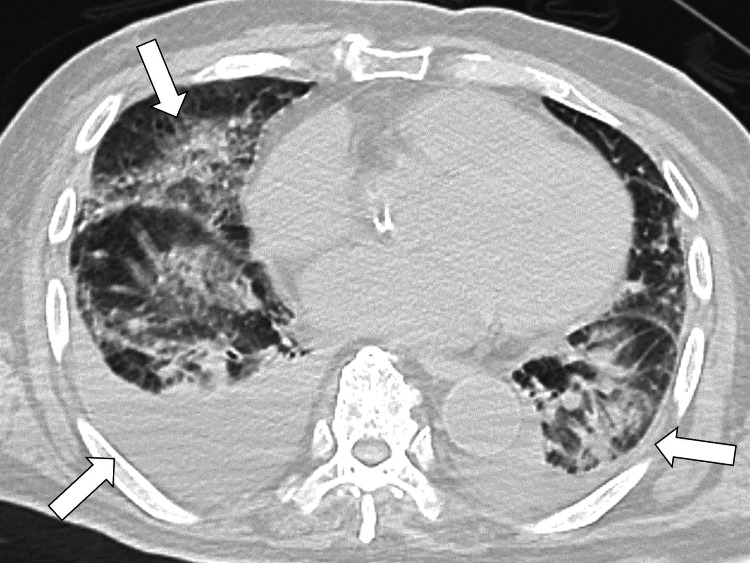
CT of the chest showing infiltrative shadows and pleural effusions in bilateral lungs (white arrows) CT: computed tomography
